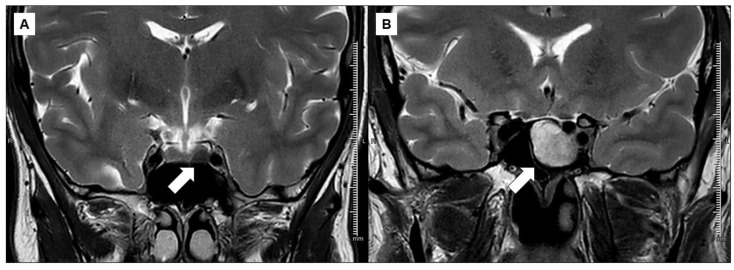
Radiological images showing two example cases. Left a markedly hypointense somatotroph adenoma in T2-weighted MRI scans (A). By way of comparison, a hyperintense somatotroph adenoma in T2-weighted MRI scans (B) is shown on the right.
